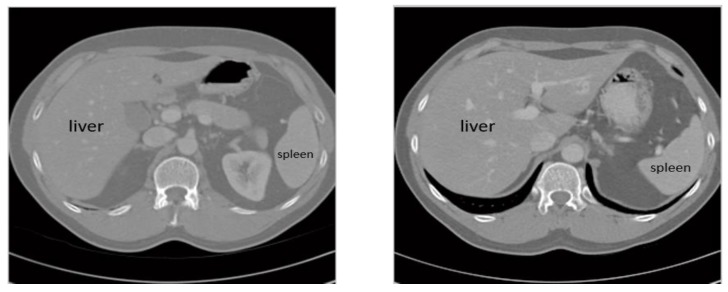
Example of the similarity in gray levels between the liver and the spleen in computed tomography (CT) images. Imported from the Medical Image Computing and Computer Assisted Intervention (MICCAI) SLIVER07 workshop datasets [24,25].
